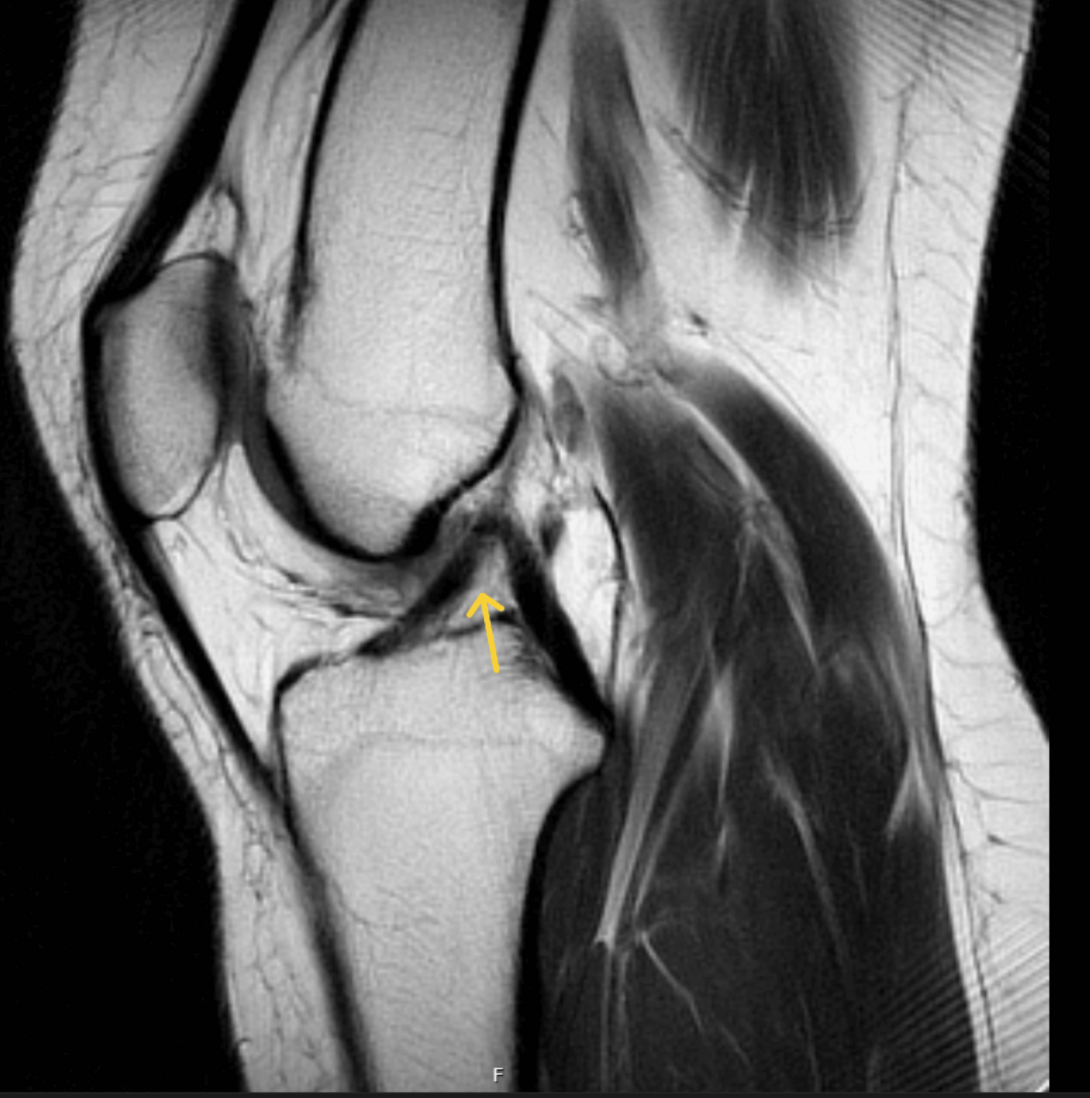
Sagittal proton density MRI of the right knee demonstrating an intact ACL (yellow arrow) ACL: anterior cruciate ligament
